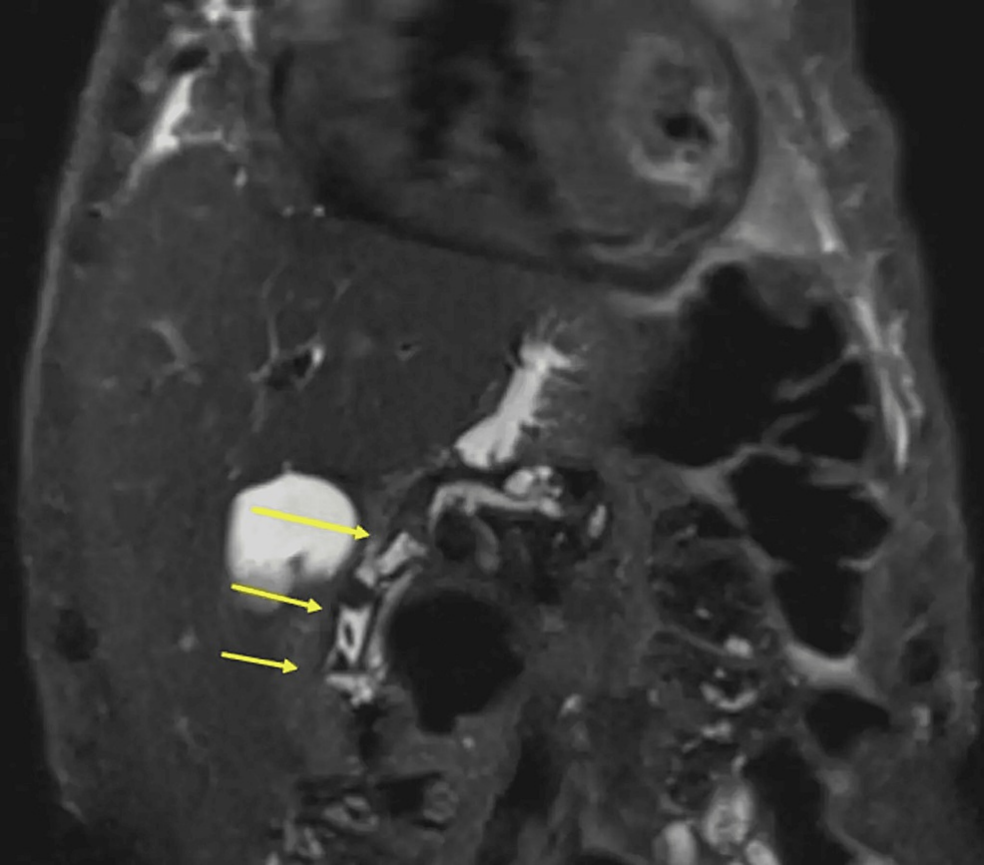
MRCP (coronal T2-weighted) HASTE image with fat saturation demonstrating three gallstones within the common bile duct (yellow arrows) MRCP: magnetic resonance cholangiopancreatography; HASTE: half fourier single-shot turbo spin-echo
